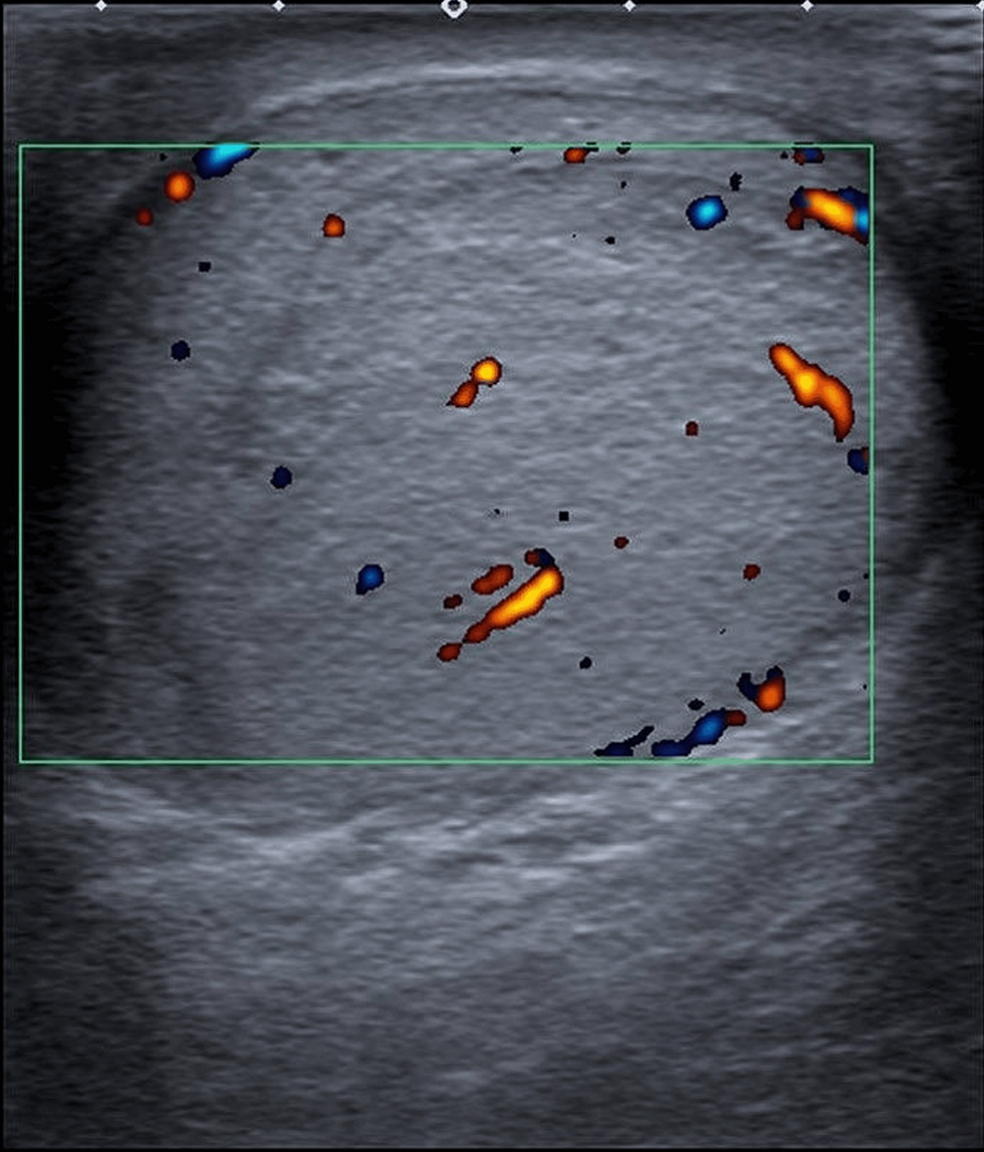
Doppler USS of the left testis showing enlargement and heterogenous echotexture.
